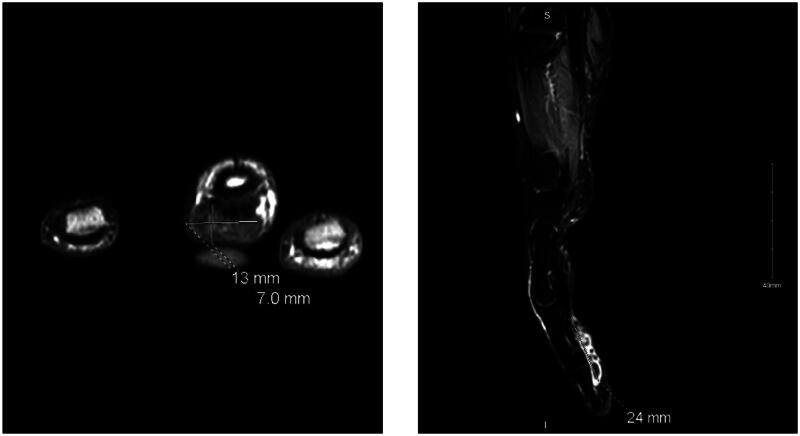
MRI of the right Middle finger.
